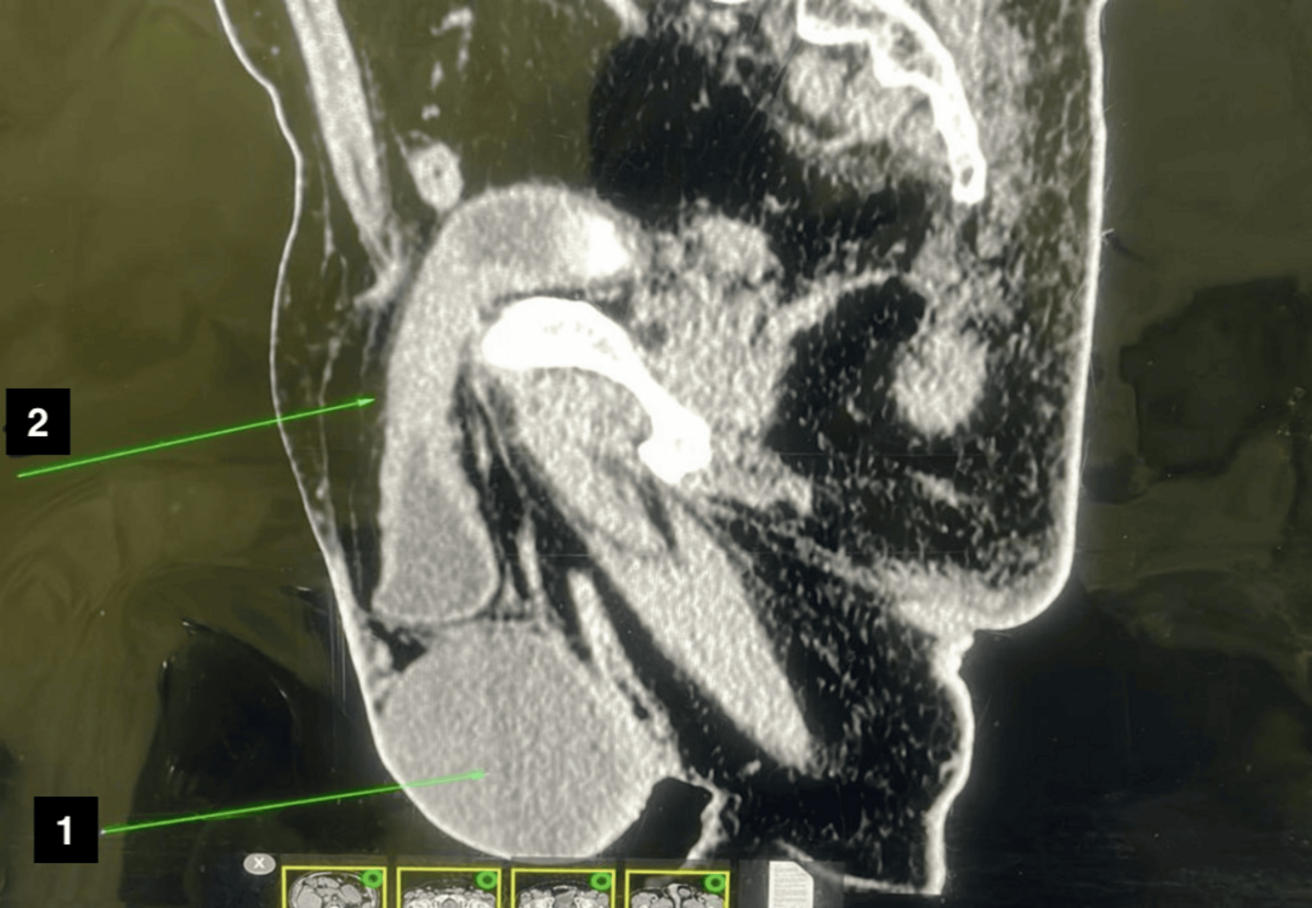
CT report showing a herniated urinary bladder and hydrocele in sagittal view; arrow 1 indicates a hydrocele sac; arrow 2 indicates an irreducible urinary bladder
